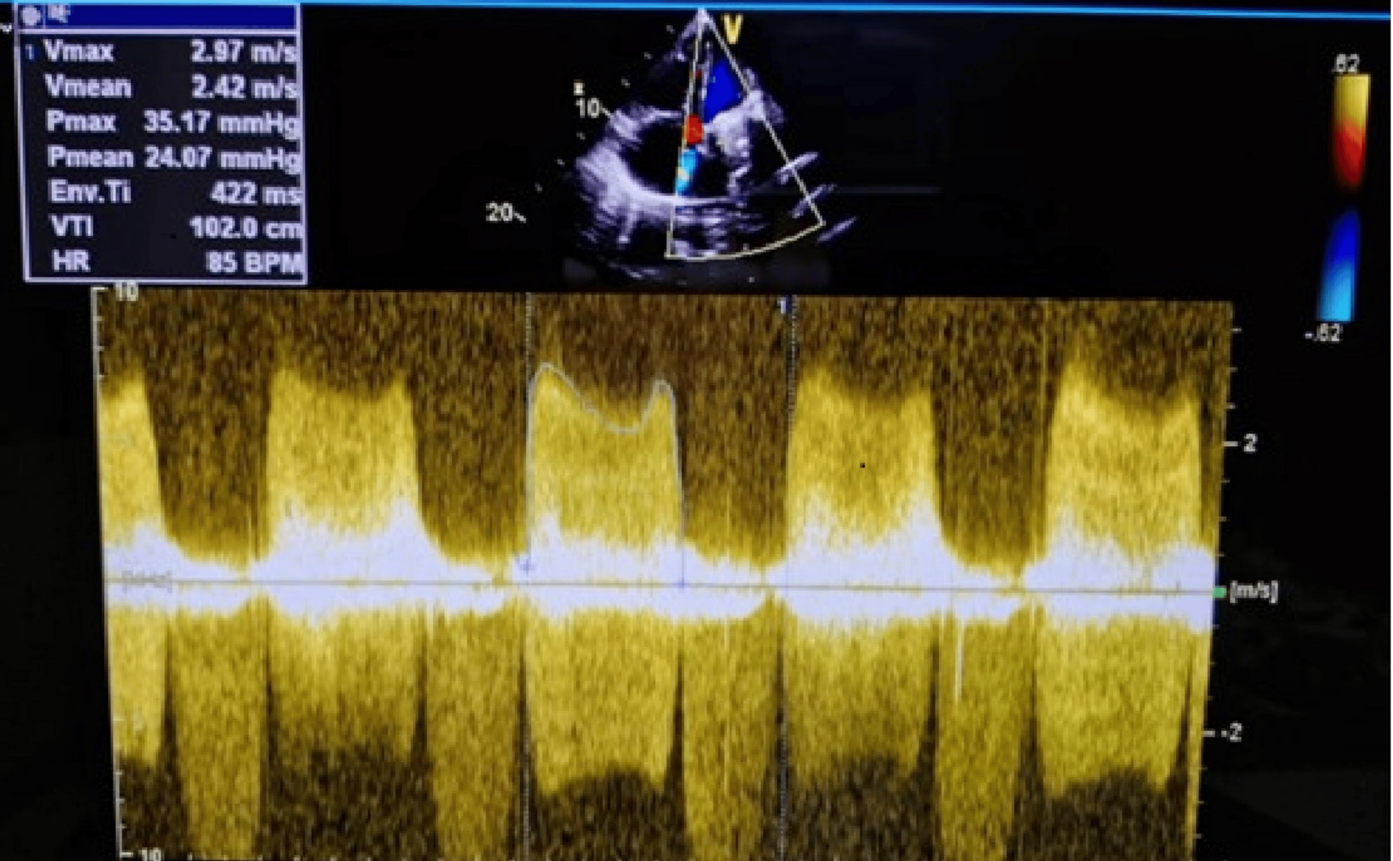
Continuous wave Doppler ultrasound during first presentation Continuous wave Doppler showing severely elevated transmitral gradients (Pmax 35.17 mmHg, Pmean 24.07 mmHg), indicating obstruction of the prosthetic valve. Pmax - peak gradient; Pmean - mean gradient
